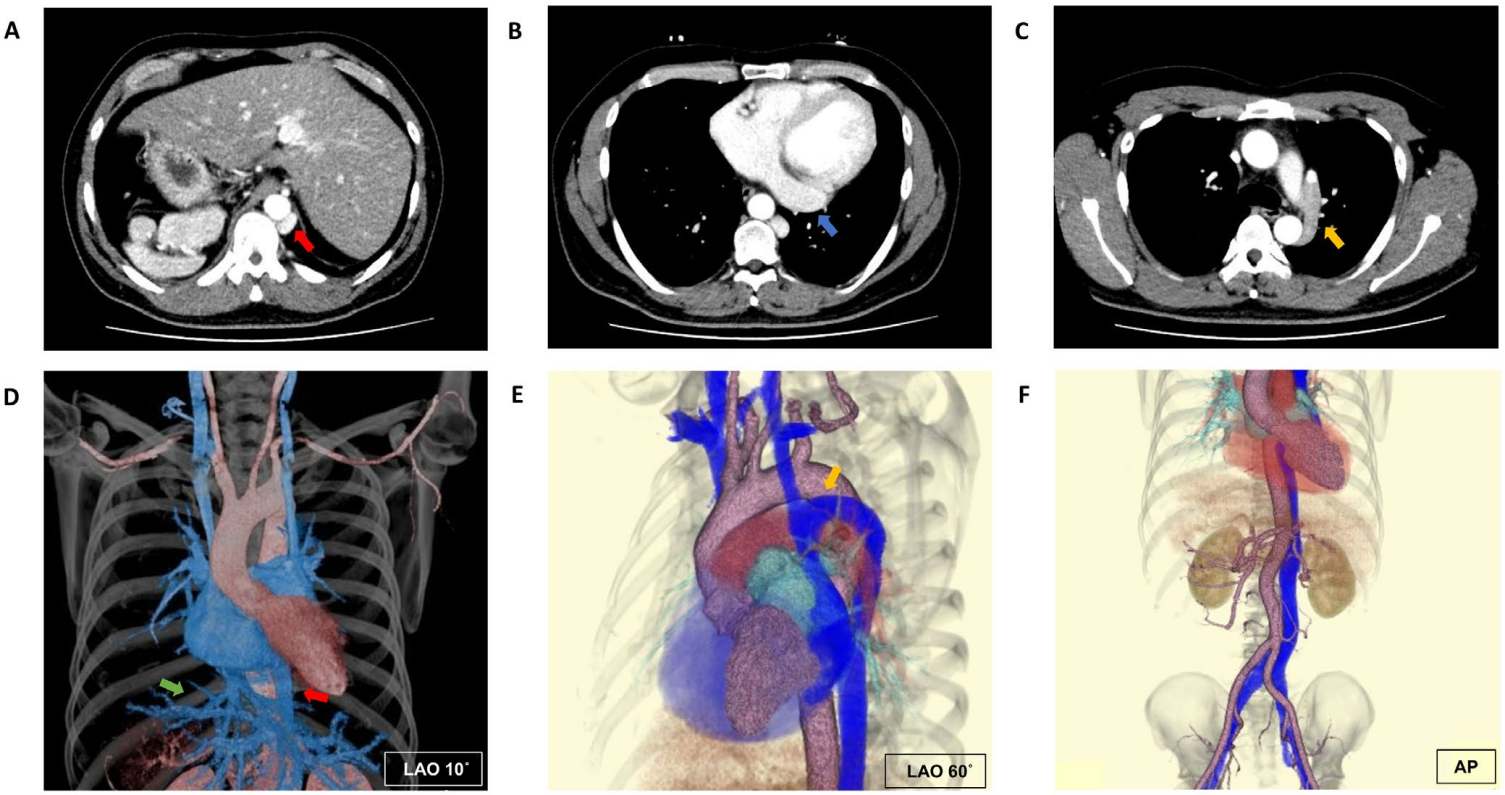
Cardiovascular anatomy. Cross‐sectional views (A–C) and volume‐rendered images (D–F) of the preprocedural computed tomography. Red, blue, yellow, and green arrows indicate interrupted inferior vena cava, persistent left superior vena cava, an azygos continuation to persistent left superior vena cava, and hepatic vein draining into the right atrium, respectively. AP, anteroposterior; LAO, left anterior oblique.
